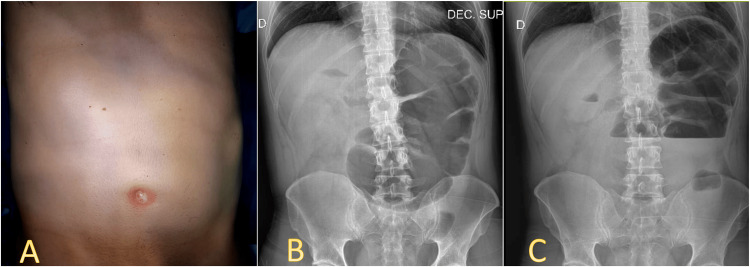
(A) Abdominal distention on physical examination. A plain radiograph (B) on the decubitus position, with a markedly dilated cecum located in the left hemiabdomen, (C) In the erect position with fluid levels present.
